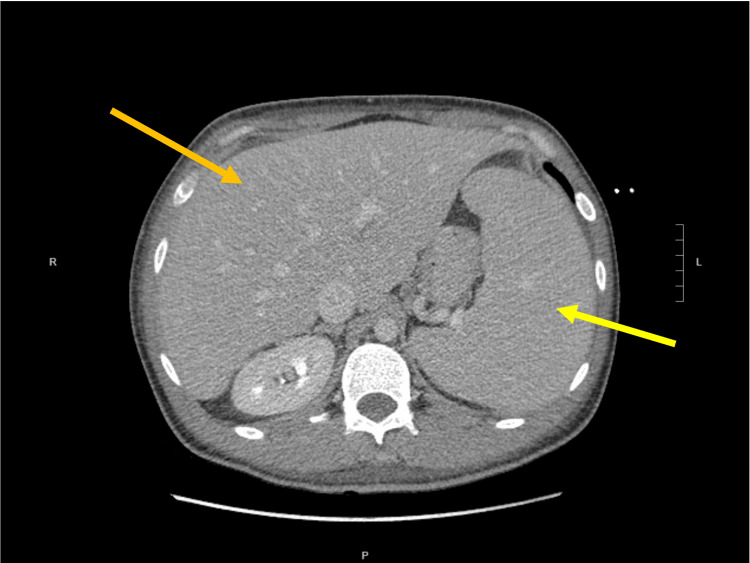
A CT scan of the abdomen and pelvis demonstrating hepatomegaly (orange arrow) and splenomegaly (yellow arrow) The spleen measured 16.2 cm in anterior-posterior diameter.
